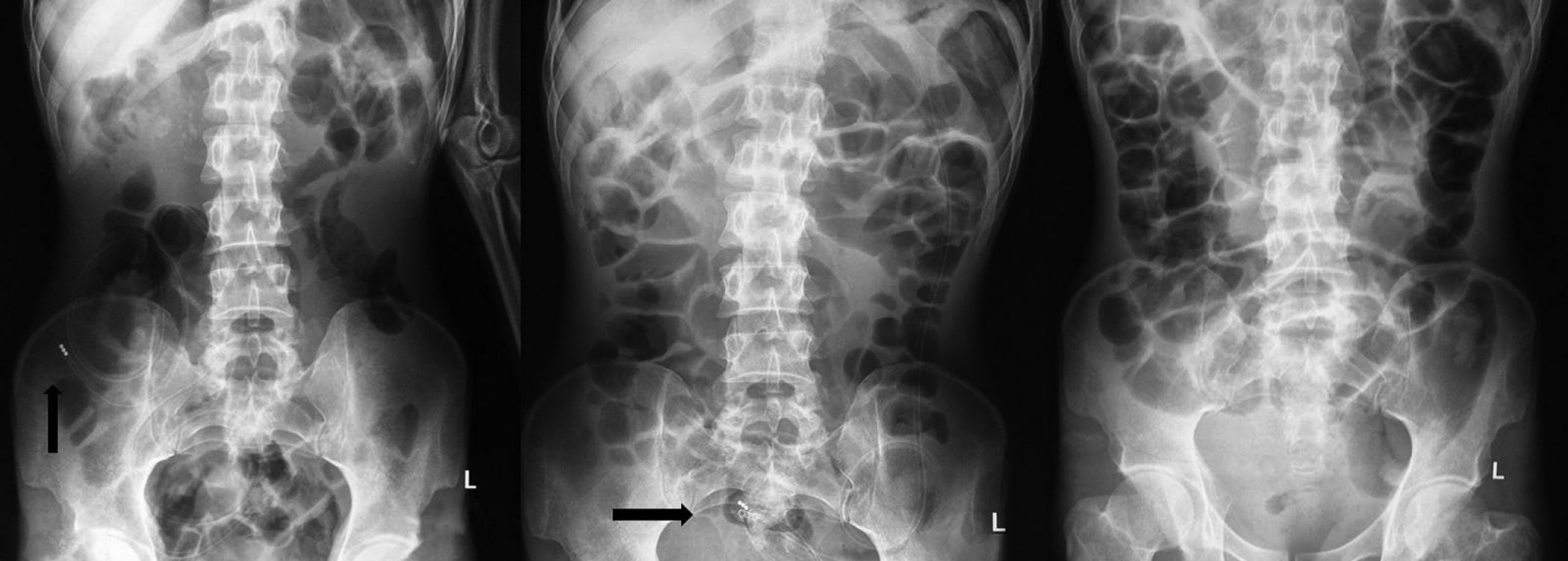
X-ray view of patient before discharge
